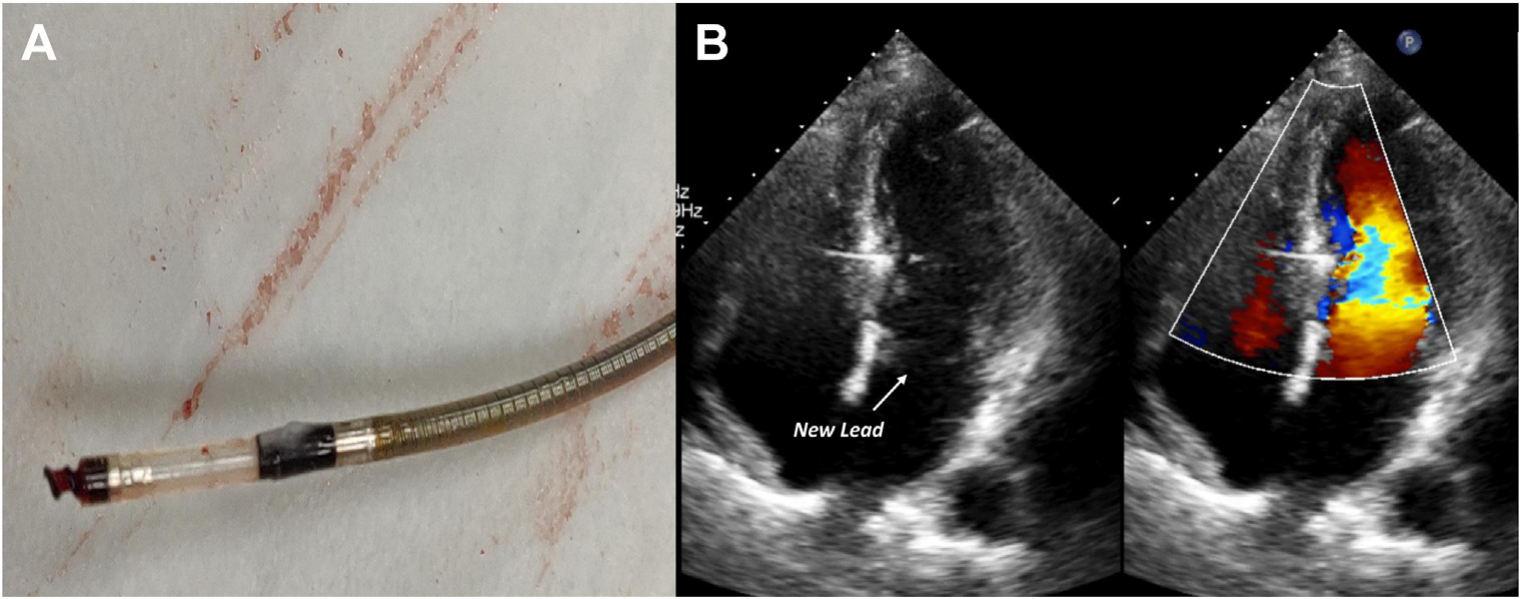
Lumenless pacing lead extraction. A: Lead extracted after 2 years en masse without damaging the helix. B: Echocardiography showing no significant septal defect after extraction of 1.4-mm sized lumenless lead. Note the position of the new lumenless lead at the midseptum reaching left ventricular subendocardium.
